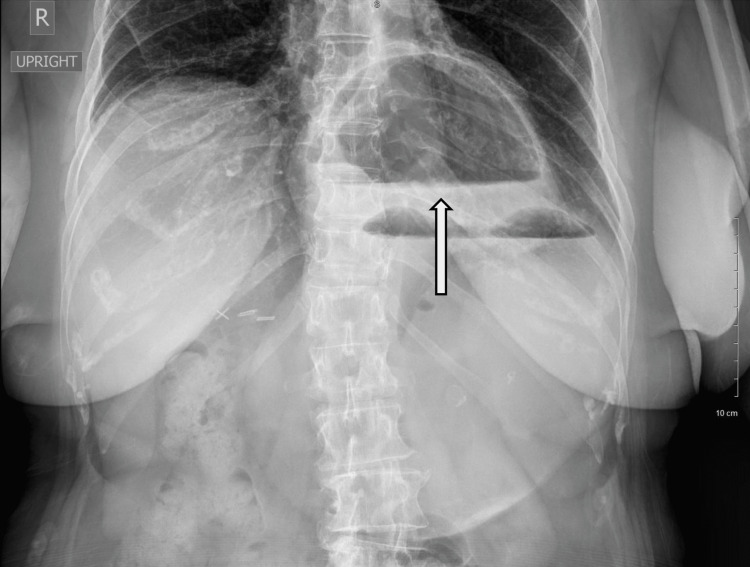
Upright radiograph Abdominal and chest radiograph in posteroanterior view shows large air-fluid level mass at the left upper quadrant, indicating a hiatal hernia (arrow). There is no sign of pneumoperitoneum, indicating no evidence of perforation.
